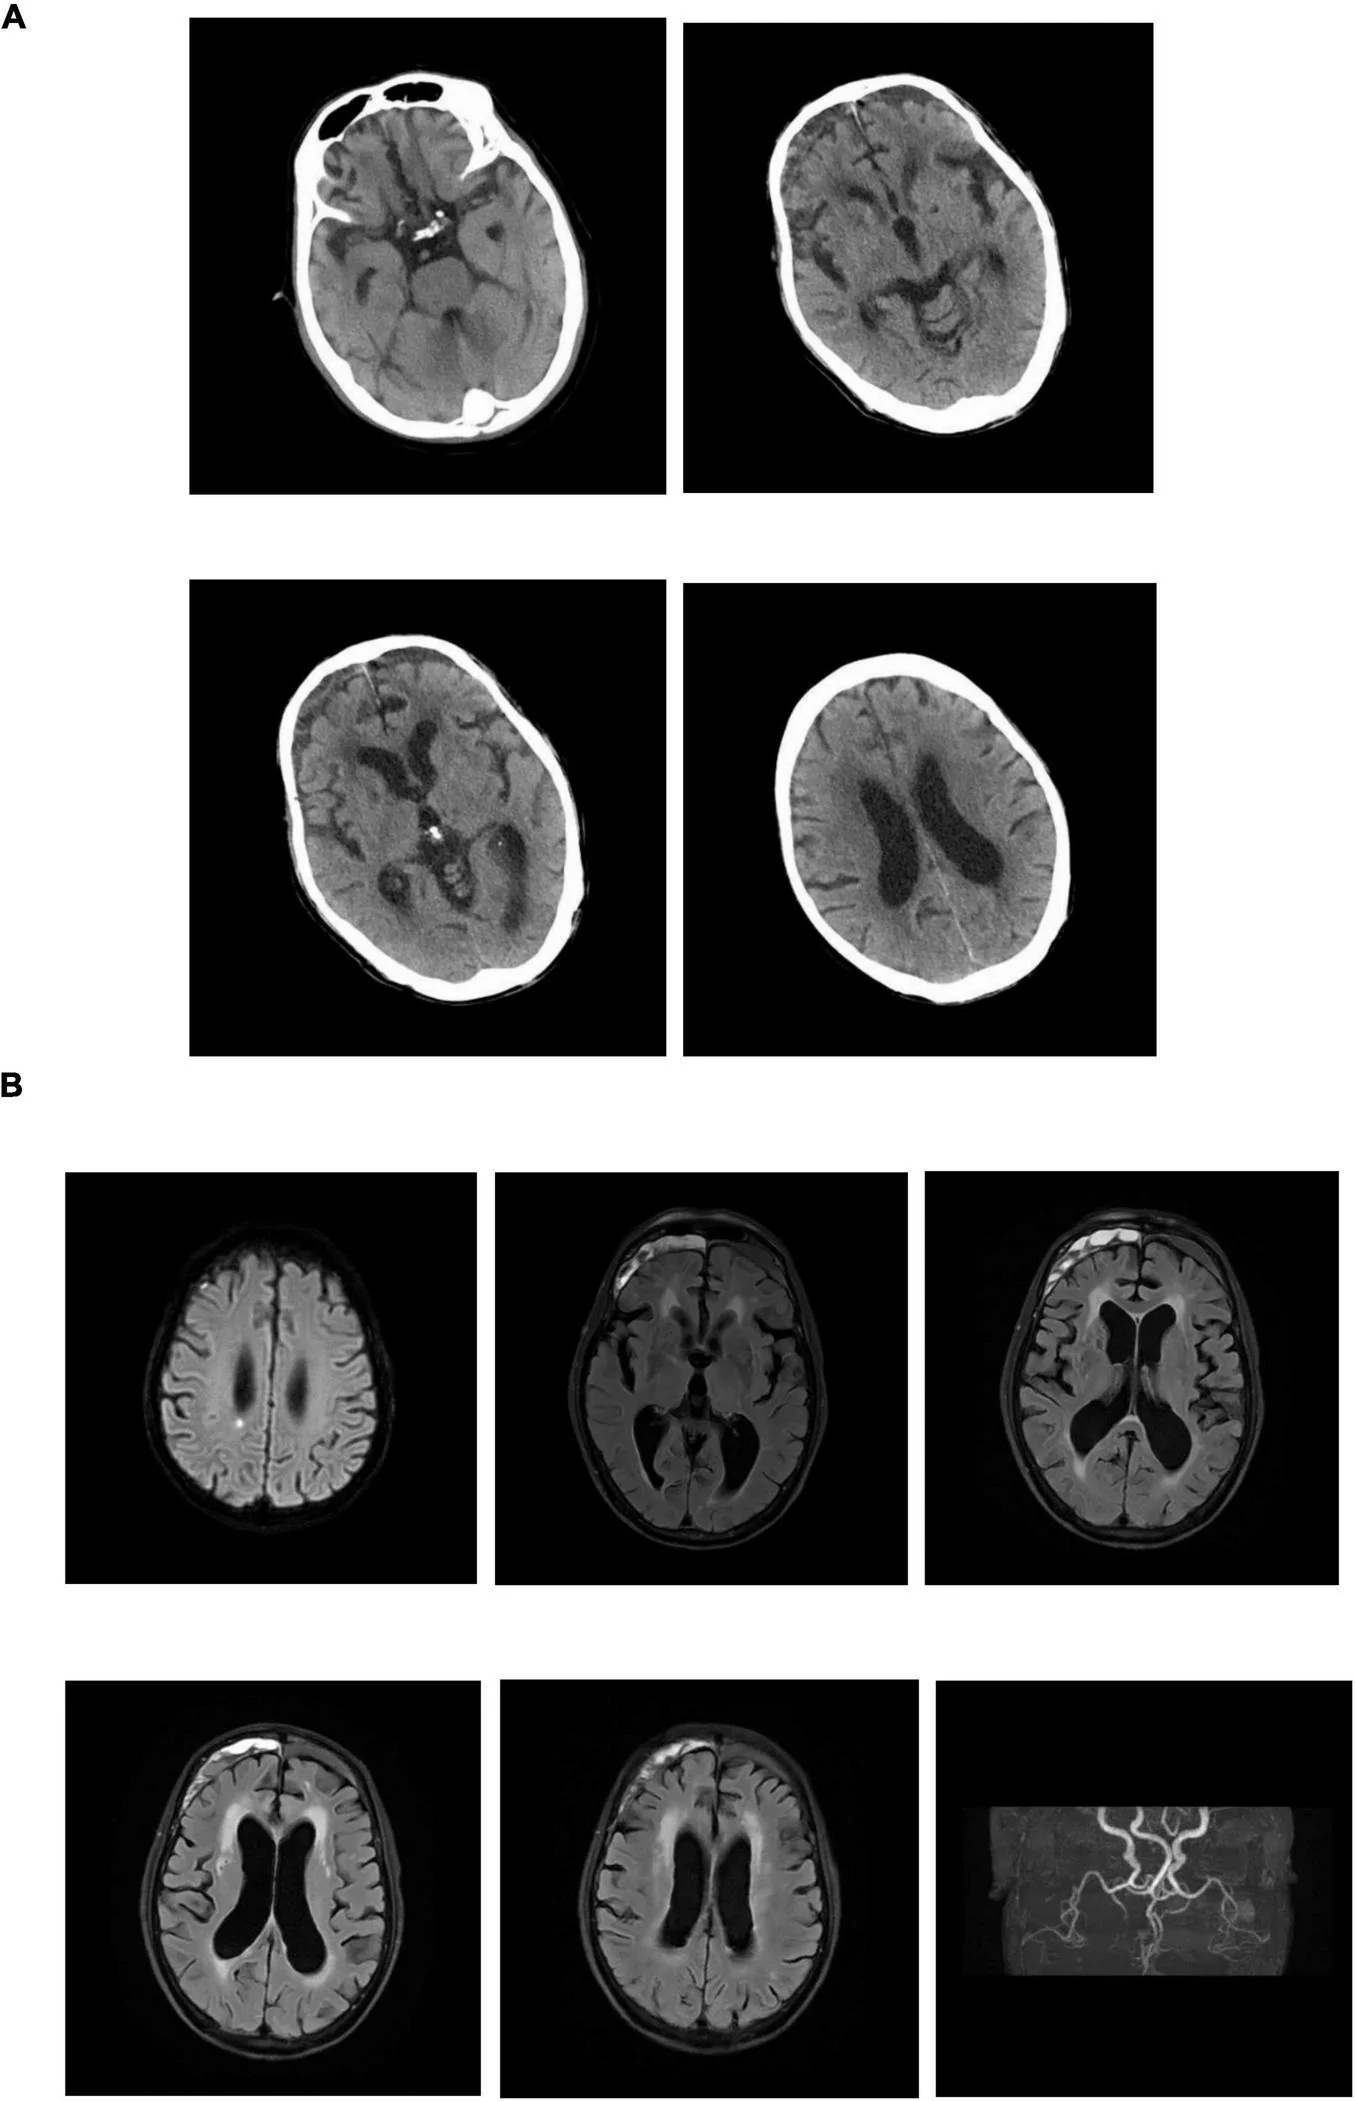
Neuroimaging findings after traumatic brain injury. (A) Non-contrast head computed tomography showed bilateral frontal subdural hematomas in the subacute-to-chronic stage, a small subarachnoid hemorrhage, a right occipital fracture, chronic lacunar infarcts in both basal ganglia, white matter demyelination, and age-related cerebral changes. (B) Brain magnetic resonance imaging with diffusion-weighted imaging and magnetic resonance angiography on a 3.0-T scanner showed a punctate diffusion-restricted lesion in the splenium of the corpus callosum, suspected contusion or acute lacunar infarction; encephalomalacia in the left temporal and right frontal lobes; multiple chronic lacunar infarcts involving the corpus callosum, bilateral basal ganglia, frontal and parietal lobes, centrum semiovale, and periventricular regions; ischemic demyelination; mild bilateral frontal subdural blood/effusion; and cerebral atherosclerosis.
